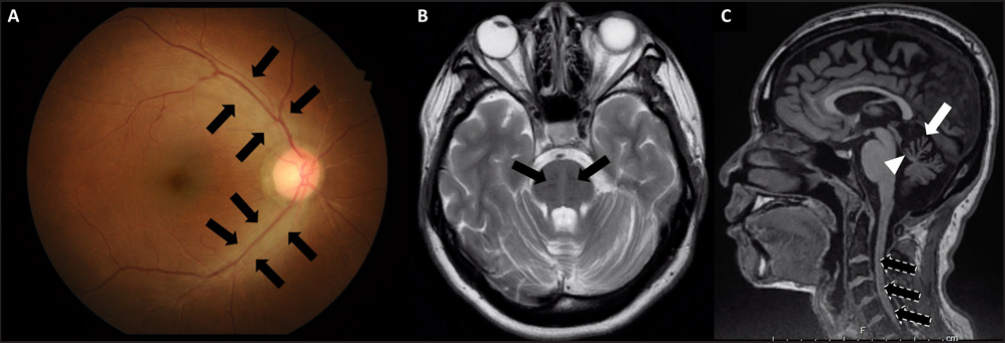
Funduscopic and Neuroimaging findings of the patient. (1A) Hypermyelinated nerve fibers radiating from the optic disc (black arrow); (1B) T2-weighted brain MRI showed multiple perpendicular linear hypointensities in pontine parenchyma (black arrow) and (1C) Superoanterior vermian (white arrow) and superior cerebellar peduncle (white arrow head) atrophy, as well as mild atrophy of the cervical spinal cord (black arrow with dash outline).
